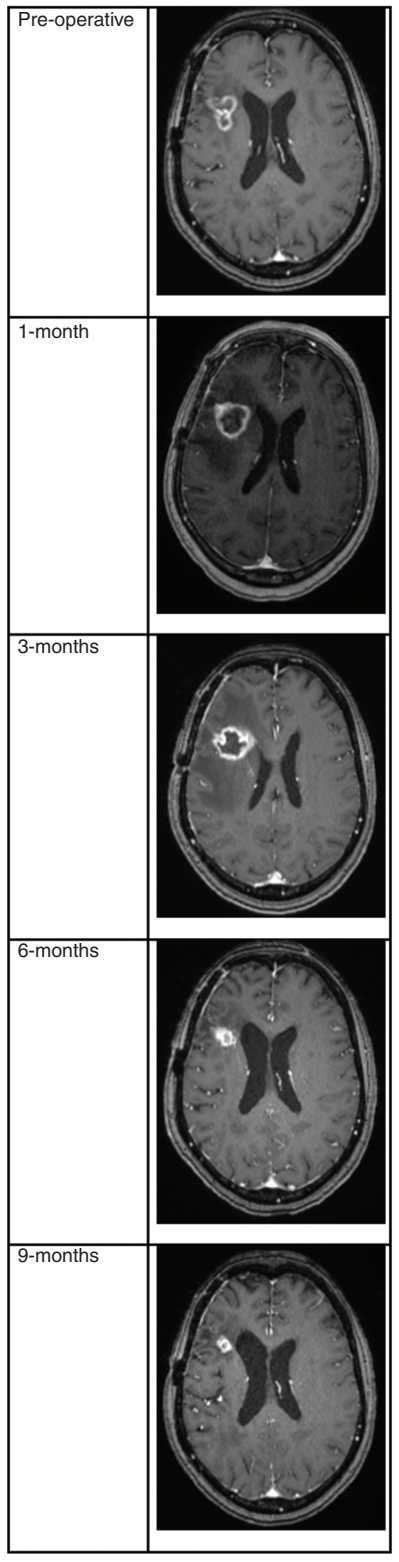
T1-weighted magnetic resonance imaging of a patient treated for radiation necrosis after SRS for metastatic lung adenocarcinoma. Imaging progression shows typical evolution of effective laser interstitial thermal therapy (LITT) ablation with initial contrast-enhancing thin-rimmed expansion followed by contraction over the following months. SRS, stereotactic radiosurgery.
